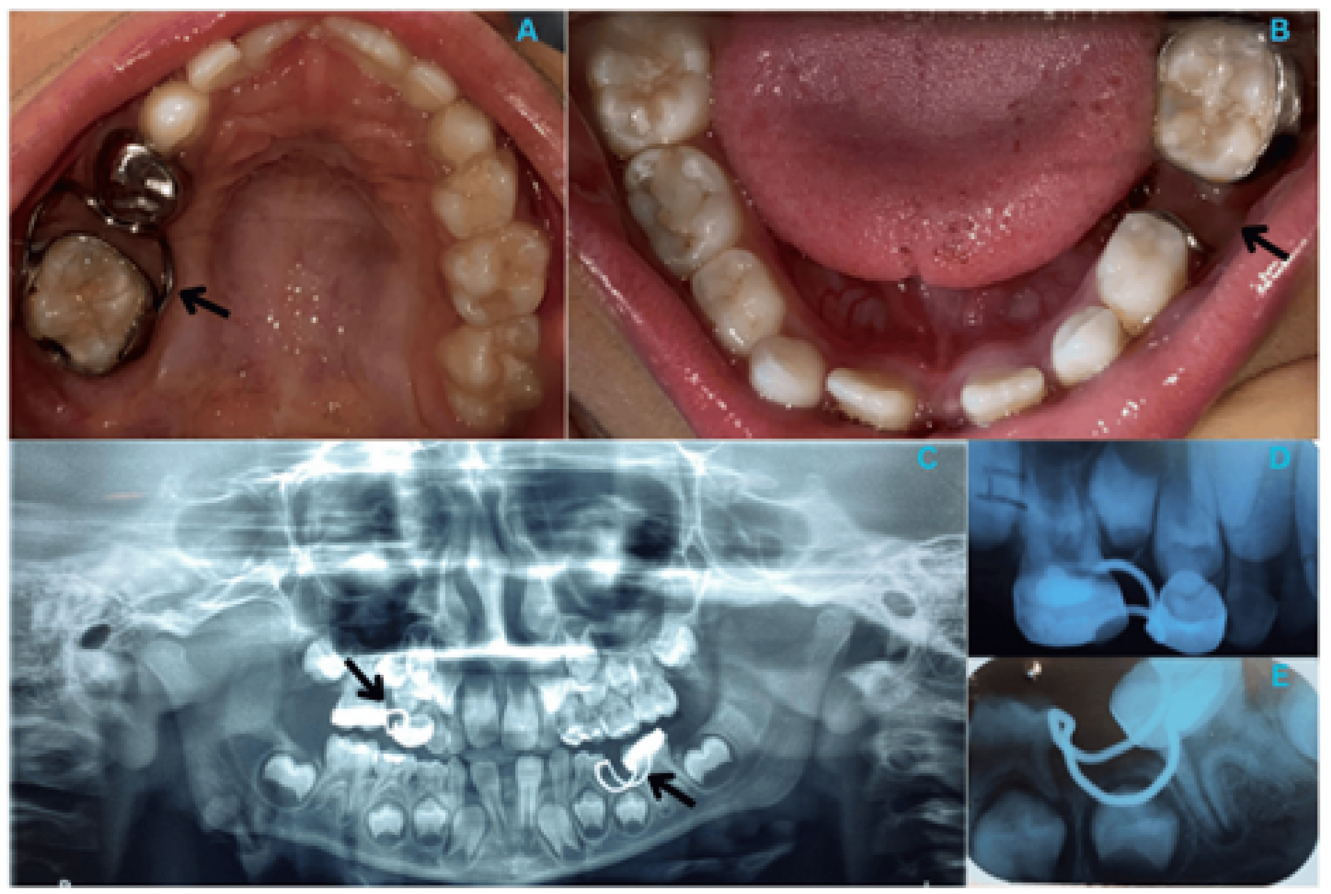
Postoperative Photograph and Radiographs (A) Postoperative maxillary occlusal view. (B) Postoperative mandibular occlusal view. (C) Orthopantomogram. (D) Intraoral periapical radiograph in relation to tooth 55. (E) Intraoral periapical radiograph in relation to tooth 75.
